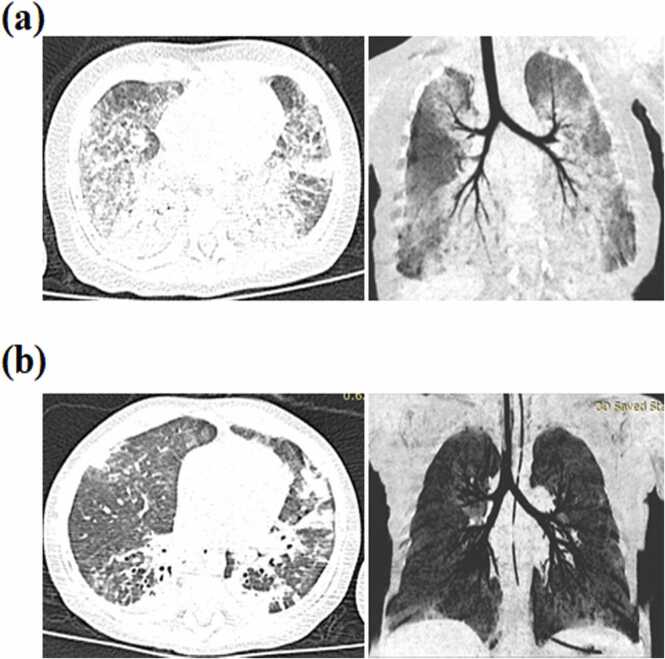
Lung windows from high-resolution CT scans of the patients. (a) Bilateral diffuse exudative and ground-glass opacity changes. (b) The shadow was obviously absorbed after 4 weeks.
